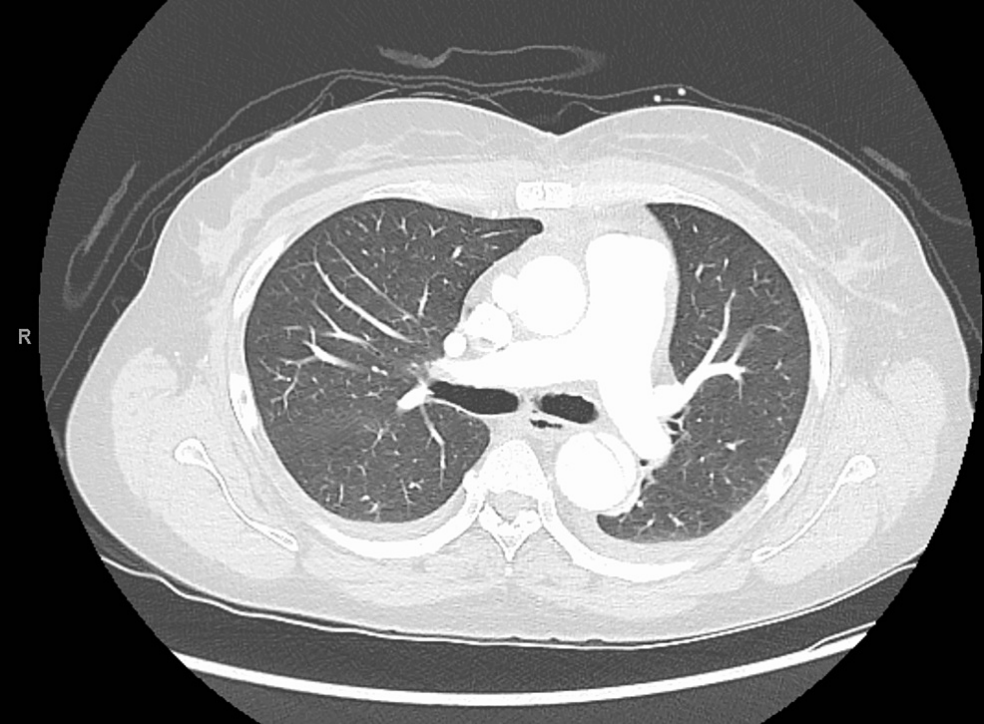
CT chest done in the emergency department showing no acute cardiopulmonary pathology
